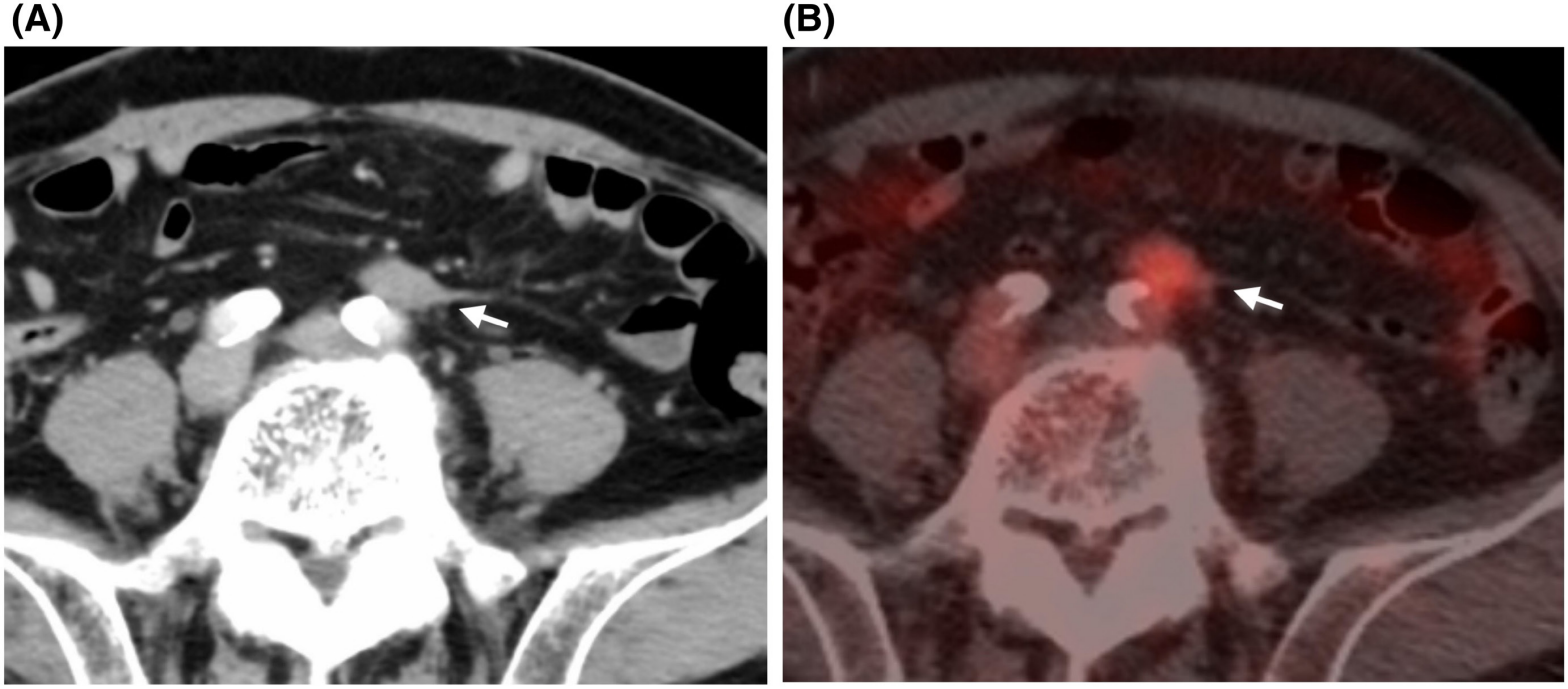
CECT scan and 18F‐FDG‐PET/CT findings in Case1. CECT scan indicates a tumor formation (21 × 12 mm) on the caudal ventral side of the left common iliac artery bifurcation (white arrow, A), corresponding to the SUVmax 5.2 tumor in the 18F‐FDG‐PET/CT scan (white arrow, B).
